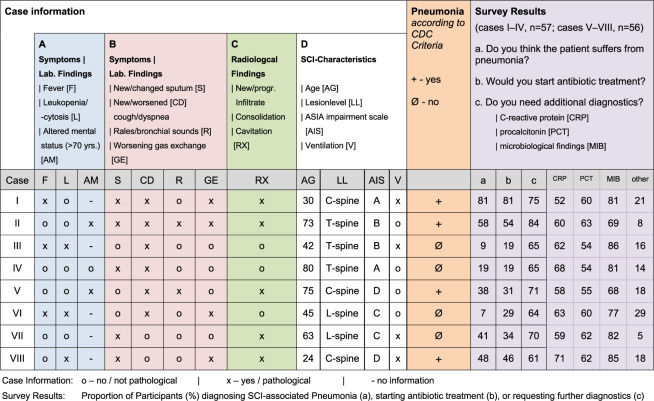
Synopsis of case vignettes and survey results. Each of the eight cases presents a pattern of clinical symptoms, laboratory values, radiological findings, and SCI characteristics (Blocks A–D). The cases were designed to either diagnose or exclude pneumonia (Block ‘Pneumonia', orange). According to CDC-criteria, at least one positive finding in Block A and with at least two positive findings in Block B in combination with one positive finding in Block C (exception: pre-existing pulmonary disease is requiring at least two repeated positive chest X-ray findings) allow for diagnosis of pneumonia. Overview of participants’ answers (relative frequencies in percent) to each of the three questions per case (Block ‘Survey Results in %’, purple).
